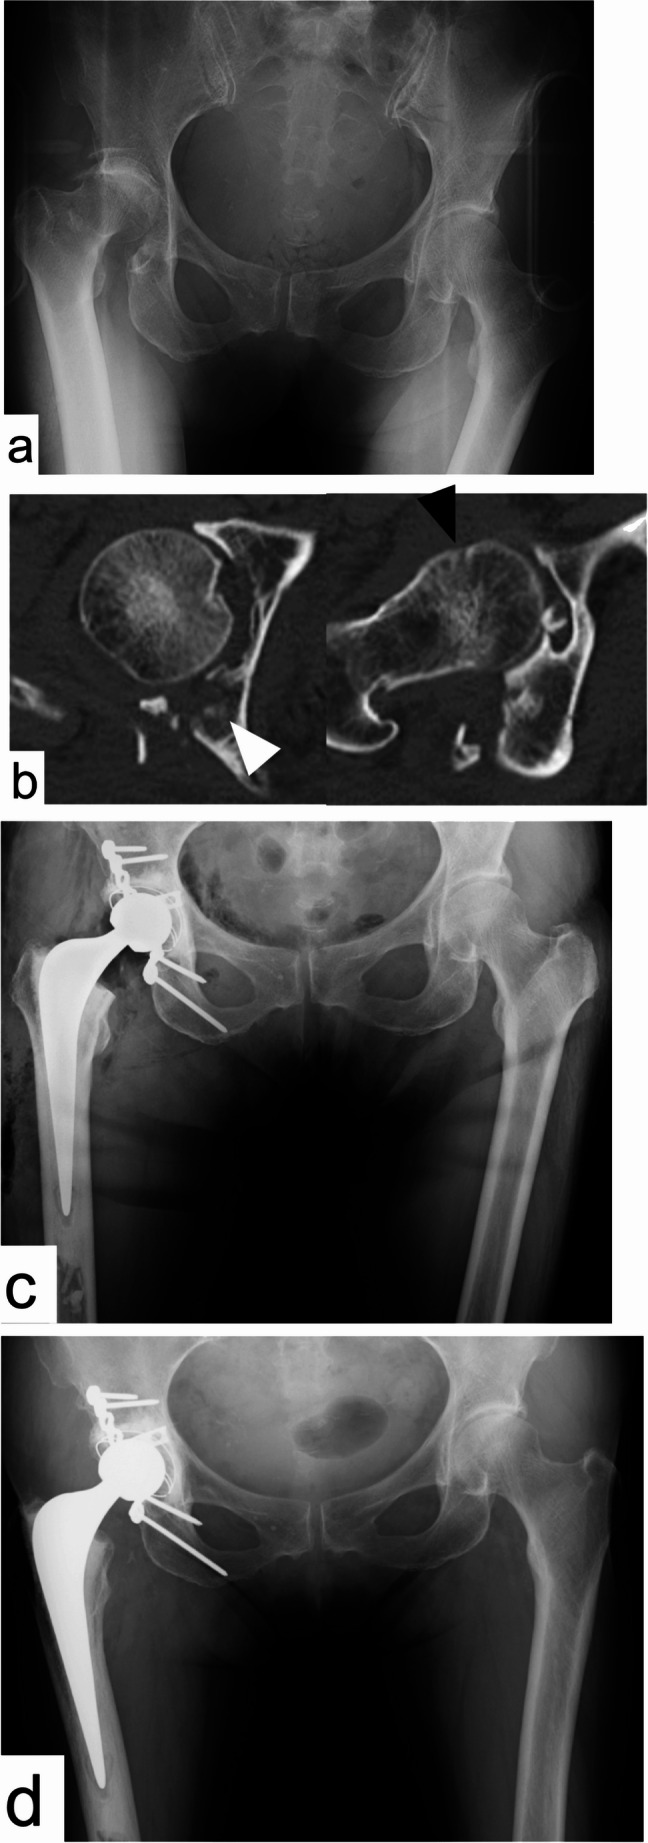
75-year-old female with posterior wall fracture and hip dislocation treated by C-THA using Kocher-Langenbech approach AP X-ray at the time of injury reveals right hip fracture dislocation CT axial view reveals marginal impaction (white arrow), posterior wall comminution, and femoral head impaction (black arrow). This case had four PPFs Postoperative AP X-ray. In this case, THA with cement cup was performed in addition to ORIF of posterior wall. HHS at 3 months was 82.9 Two-year postoperative AP X-ray
